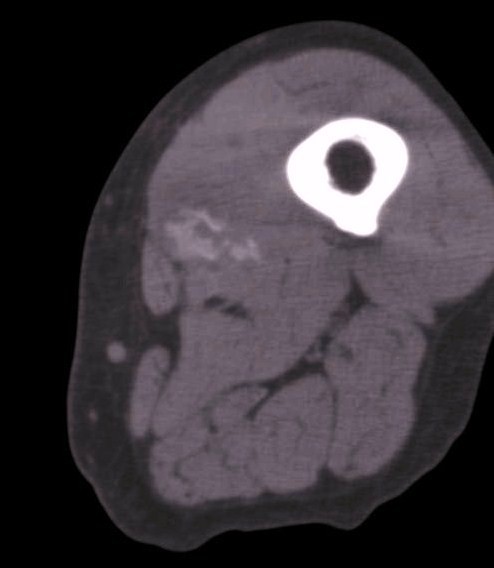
CT of the thigh shows a lesion with peripheral ossification.
